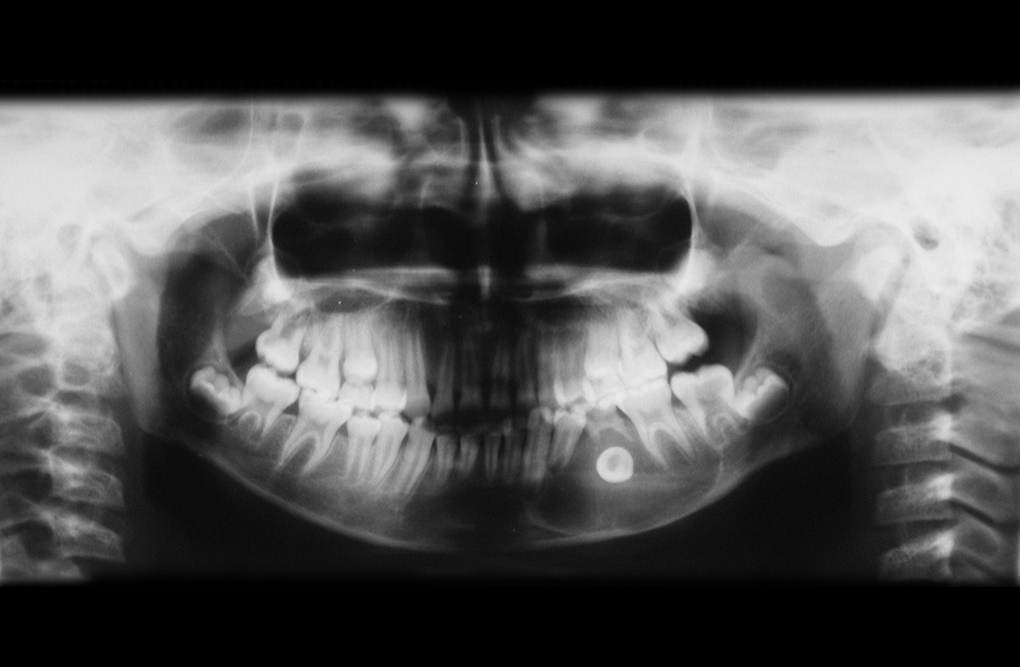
Panoramic radiograph at first examination showing unilocular radiolucent lesion, below the left second primary molar, displacing the successive permanent second premolar apically
